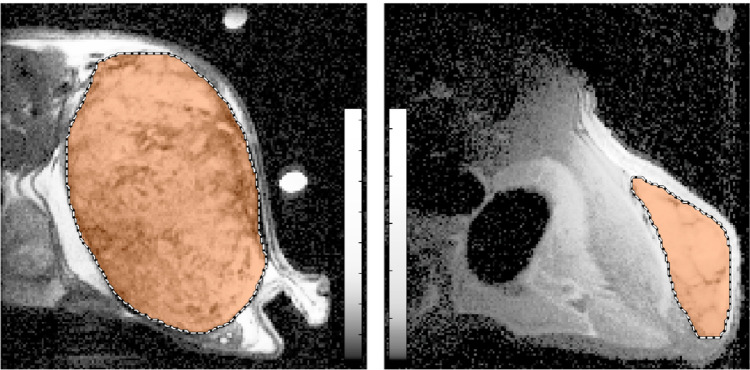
Examples of MR images and ROIs for a large tumor (left, 1.3 cm^3^) and a small tumor (right, 0.06 cm^3^). MR images are shown with histogram-equalized colormaps, and ROIs are shown as orange regions with black and white dashed borders. The large tumor is well-approximated by an ellipsoid shape, and the small tumor is less accurately represented, although this is difficult to convey in two-dimensional images.
